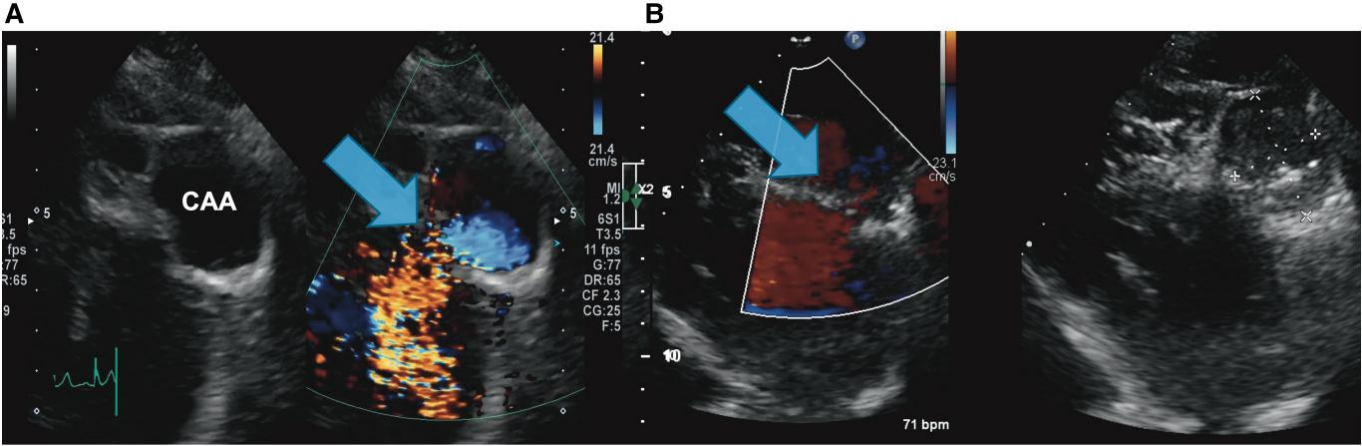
Transthoracic echocardiography (TTE) before and after the procedure. (*A*) Colour Doppler TTE before the procedure demonstrated flow from the LAD into the aneurysm sac. (*B*) Post-procedural TTE confirmed the complete cessation of flow within the aneurysm and presence of the coil. Blue arrows indicate the direction of flow into the coronary artery aneurysm (CAA).
